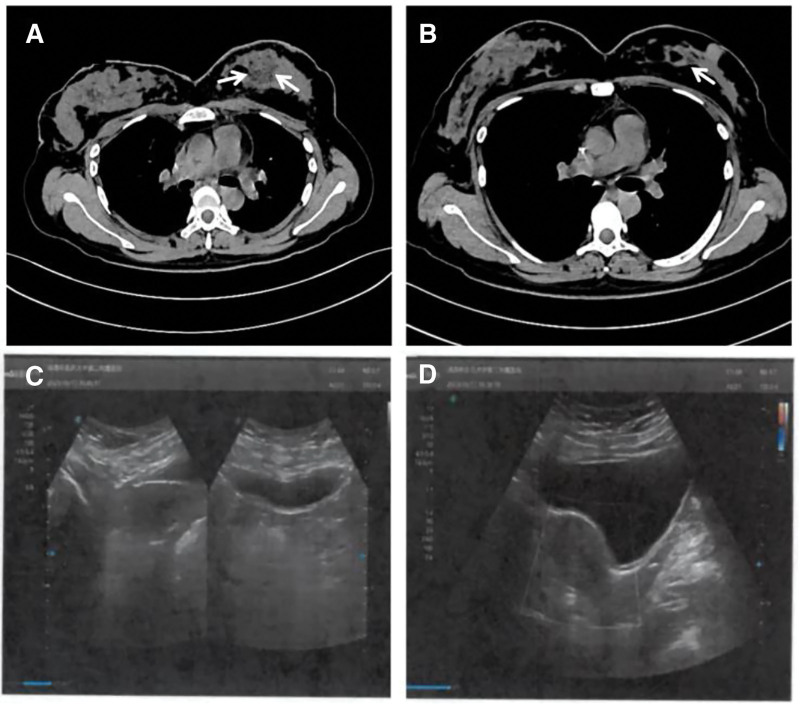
Prechemotherapy CT scan shows a circular hypodense lesion (A); postchemotherapy CT scan of the same slice (B) reveals marked reduction in the hypodense lesion, which is now difficult to discern. (C, D) Preoperative ultrasonographic images of the uterus and adnexa. No definite abnormal sonographic findings were observed in the uterus or bilateral adnexal regions. CT = computed tomography.
